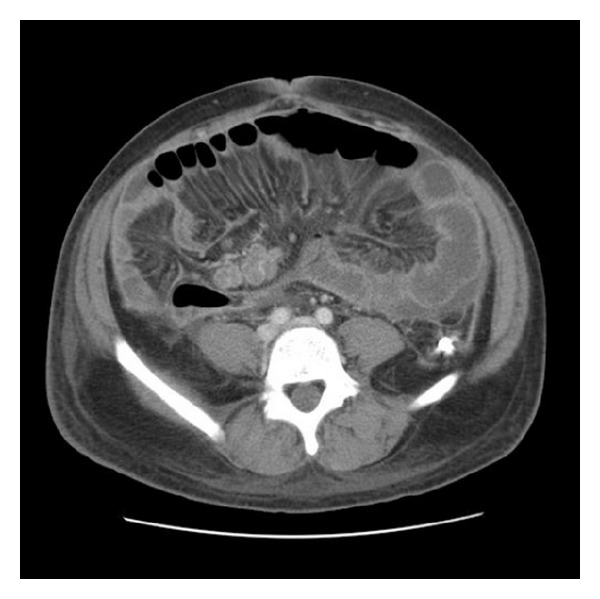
CT abdomen and pelvis revealing enlarged mesenteric lymph nodes and prominent retroperitoneal lymph nodes along with omental infiltration.
